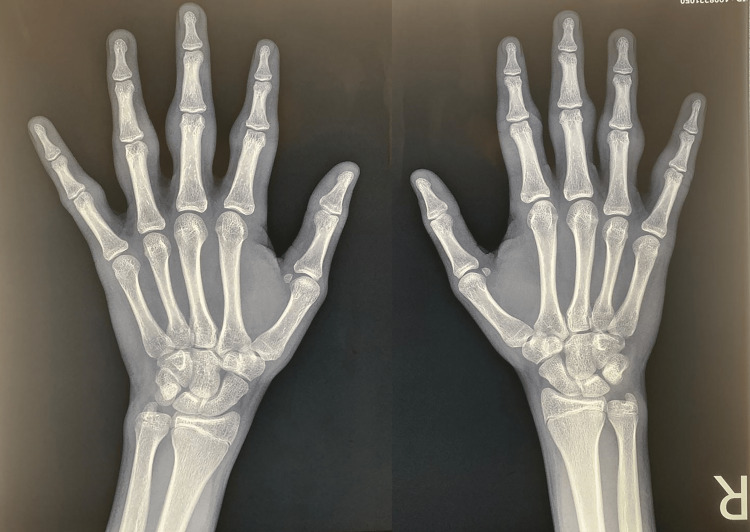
Radiograph Anteroposterior radiograph of both hands without bone and joint alteration
